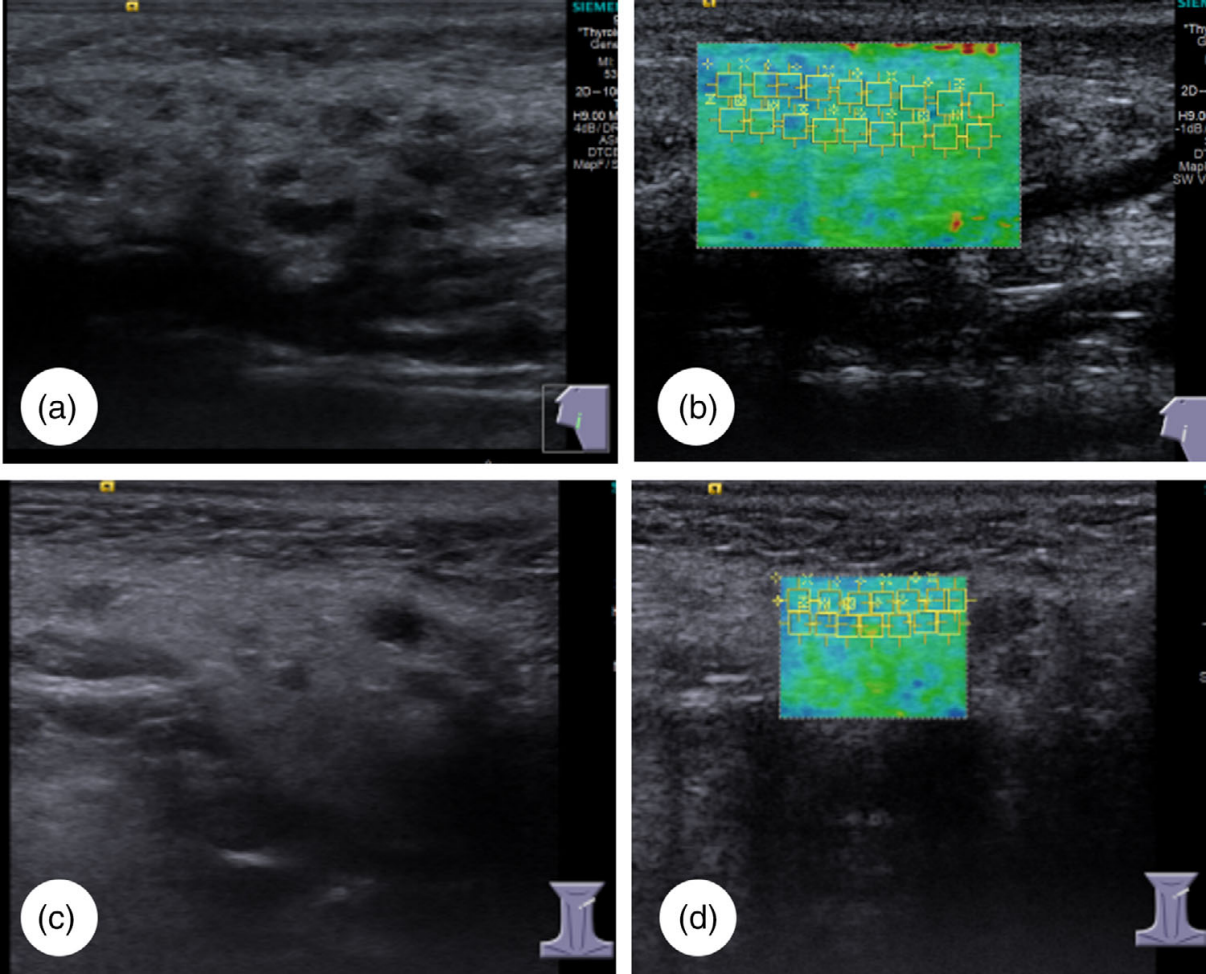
Ultrasonographic features and shear wave elastography of the parotid and submandibular glands in patients with primary Sjögren's Syndrome (pSS). (a) Two‐dimensional ultrasonography of the parotid glands. (b) The shear wave velocity (SWV) of the parotid gland is 2.485 m/s. (c) Submandibular gland two‐dimensional ultrasonography. (d) The SWV of the submandibular gland is 2.36 m/s.
